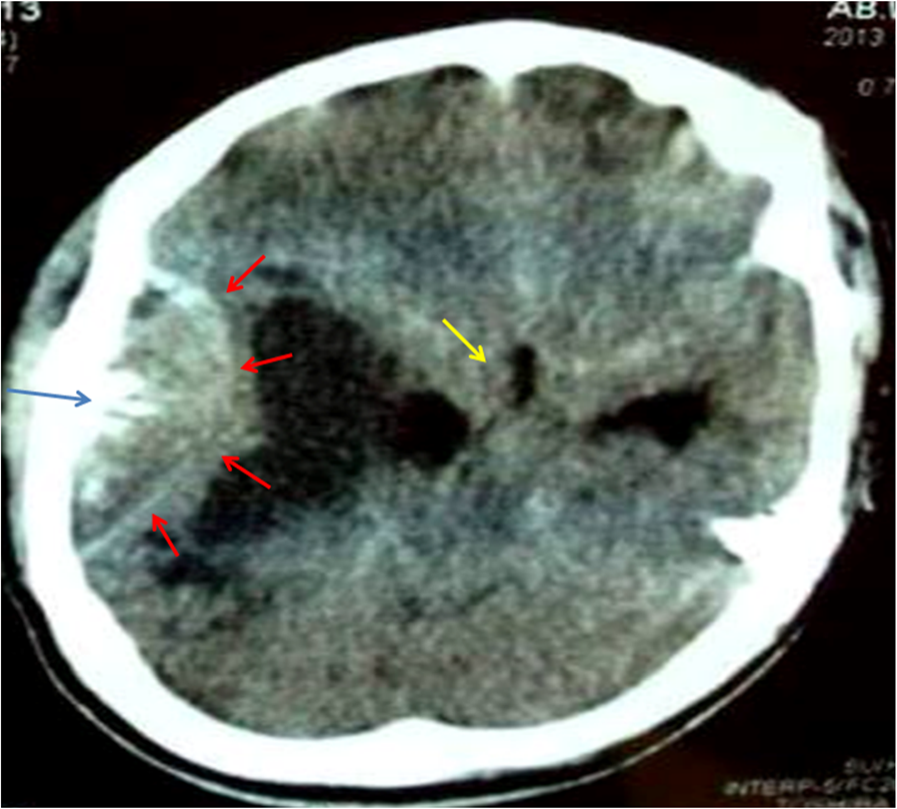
Initial non-contrasted head computed tomography (CT) scan
